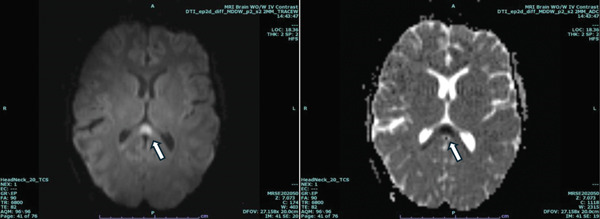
Axial MRI images of brain with arrows showing cytotoxic lesion of the corpus callosum (CLOCC).
